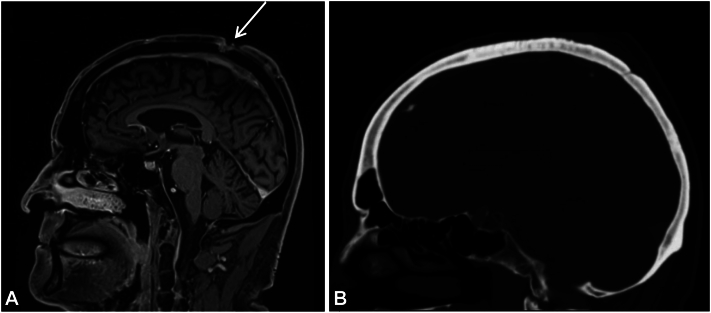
A, Magnetic resonance imaging head (T1, sagittal). *Arrow* indicates area of outer calvarial erosion of skull vertex. B, Computed tomography head (sagittal) 7 weeks earlier without evidence of calvarial erosion.
